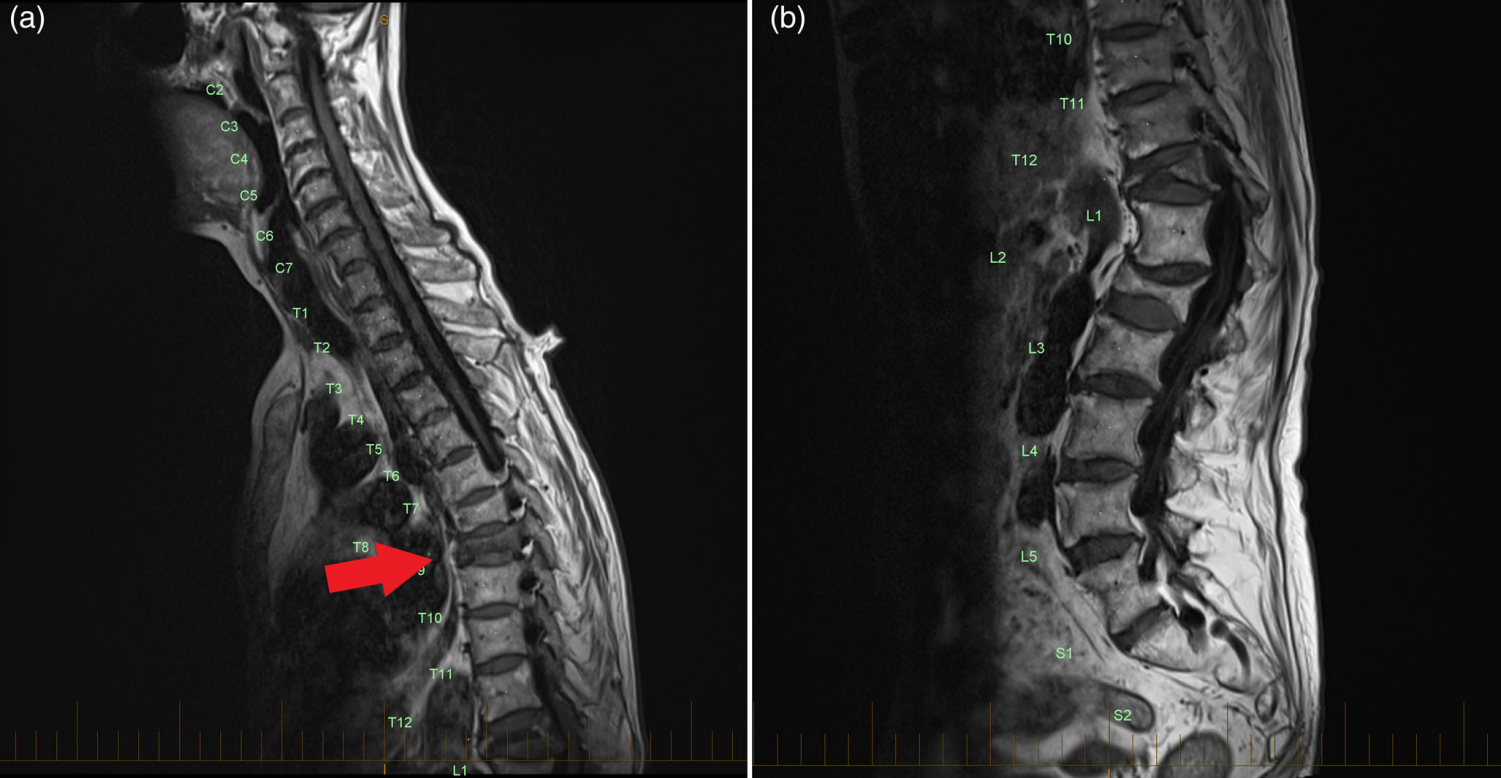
T_1_‐weighted MRI imaging of the thoracic (*A*) and lumbar spine (*B*) showing an acute compression fracture identified in T8 (arrow) and evidence of prior vertebral compression fractures in T7, T12, and L2.
